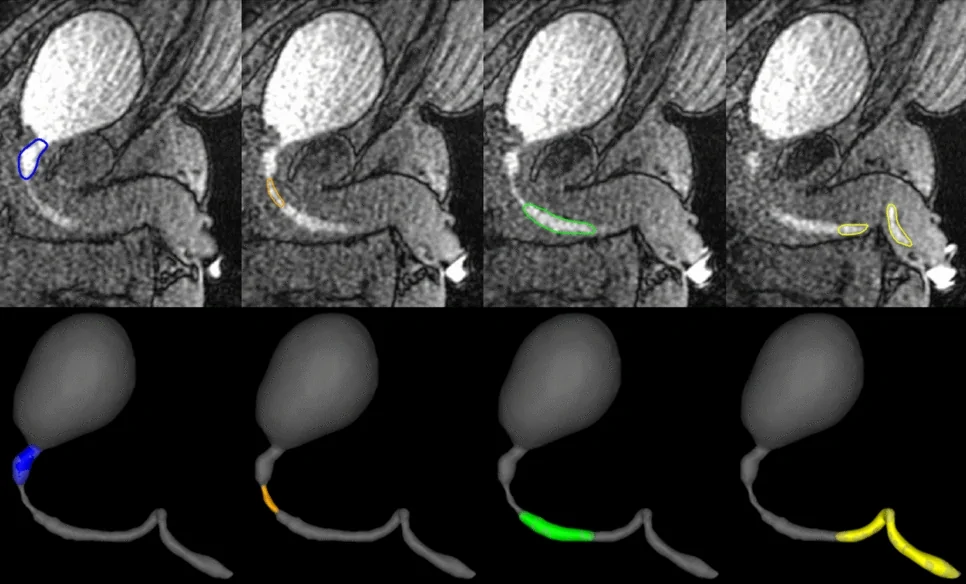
a Contour lines of the prostatic (blue), membranous (orange), bulbous (green), and penile (yellow) urethras’ segmentations overlayed on a DISCO flex oblique sagittal MR image at maximum flow rate (42.02 s). b 3D anatomical renderings of the urethral subdivisions produced from segmentations of images obtained at maximum flow. The internal urethral sphincter (IUS) located at the junction of the bladder and prostate is not specifically indicated. Note that the apparent kink in the penile urethra is due to an axial curvature and is not a luminal narrowing
